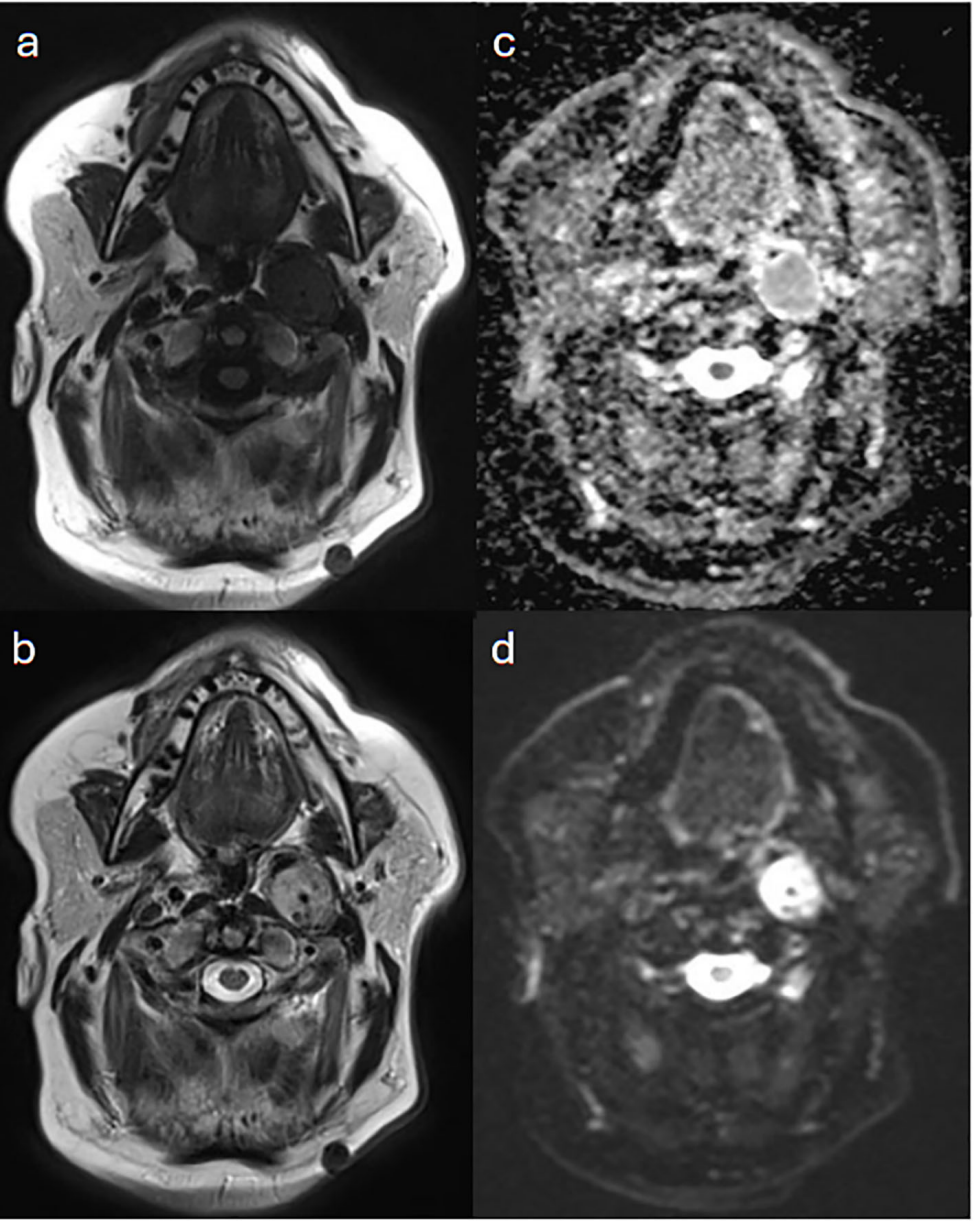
Case 1. A 2.7 cm anteroposterior enhancing carotid space lesion that contains internal flow voids on (a) T1-weighted and (b) T2-weighted MRI images. The lesion is mildly restrictive to diffusion as seen in (c) apparent diffusion coefficient (ADC map) and (d) diffusion weighted imaging, to a less extend in comparison with adjacent nodal or neuroaxis soft tissue. The lesion is superior to the carotid bifurcation, displacing both the internal and external carotid arteries anteriorly, and its superior margin is just clear of the jugular foramen. This is consistent with left vagal paraganglioma.
